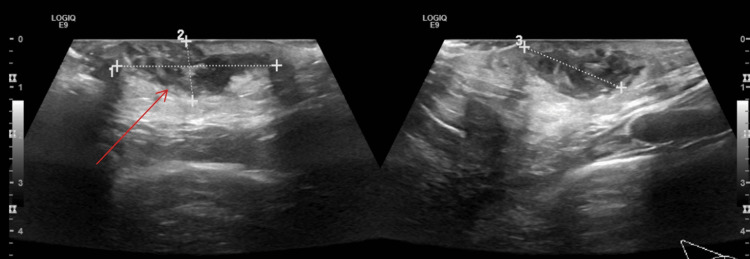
Right breast ultrasonography The arrows point at a large breast abscess measuring 33 x 12 x 22 mm.
